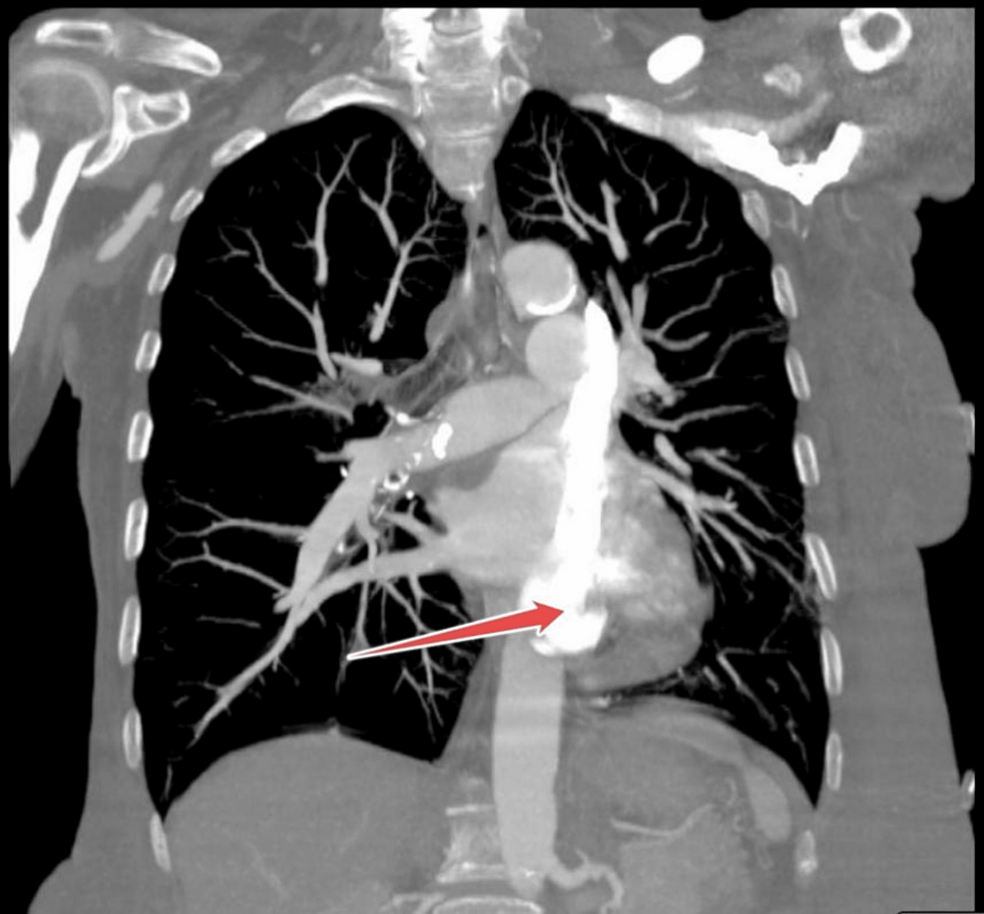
Computed tomography angiography chest indicating the left SVC entering the right atrium (the red arrow) SVC - superior vena cava
